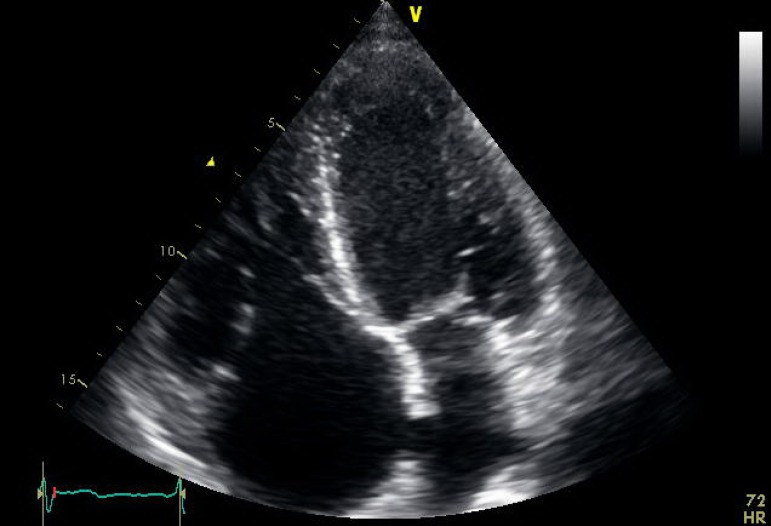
Transthoracic echocardiogram showing severely thickened tricuspid valve
